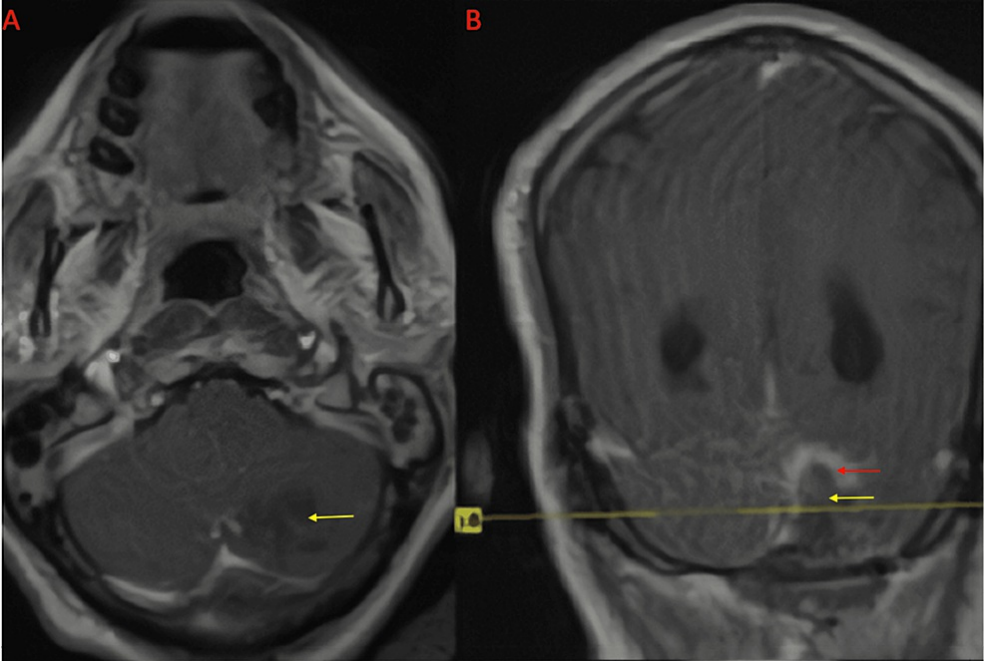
Postoperative MRI of the brain T1-weighted postcontrast imaging in the (A) axial and (B) coronal planes There is interval mass resection at the left cerebellum (yellow arrows). There is persistent enhancement along the surgical margin (red arrow, Figure 5B), which was attributed to hemostatic agents used to fill the tumor cavity during surgery MRI: magnetic resonance imaging
